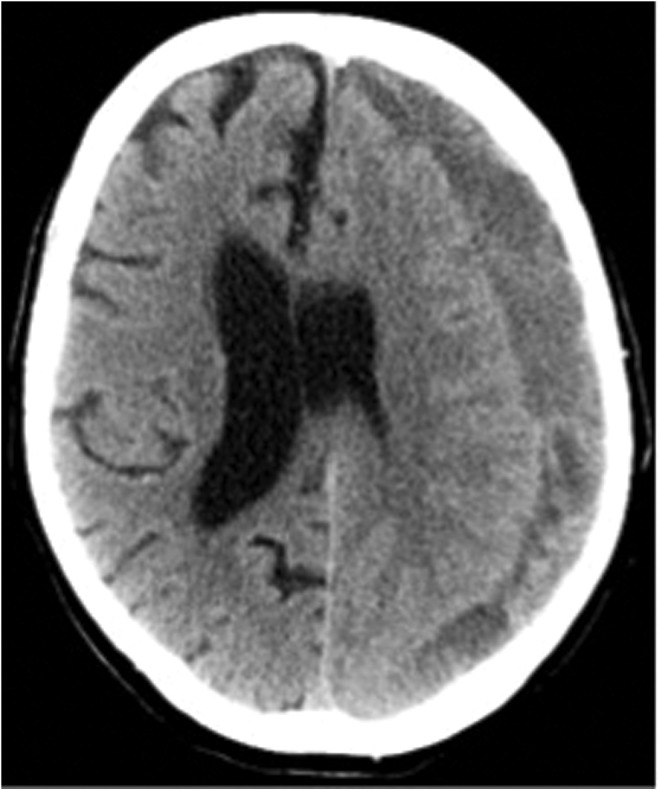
A typical appearance of a cSDH on the left side (patient P3) on a computed tomography scan, with its characteristic crescent-shaped form spanning over the coronal and lambdoid sutural margins. The hematoma is in the hypodense phase. Notice also the significant midline shift.
